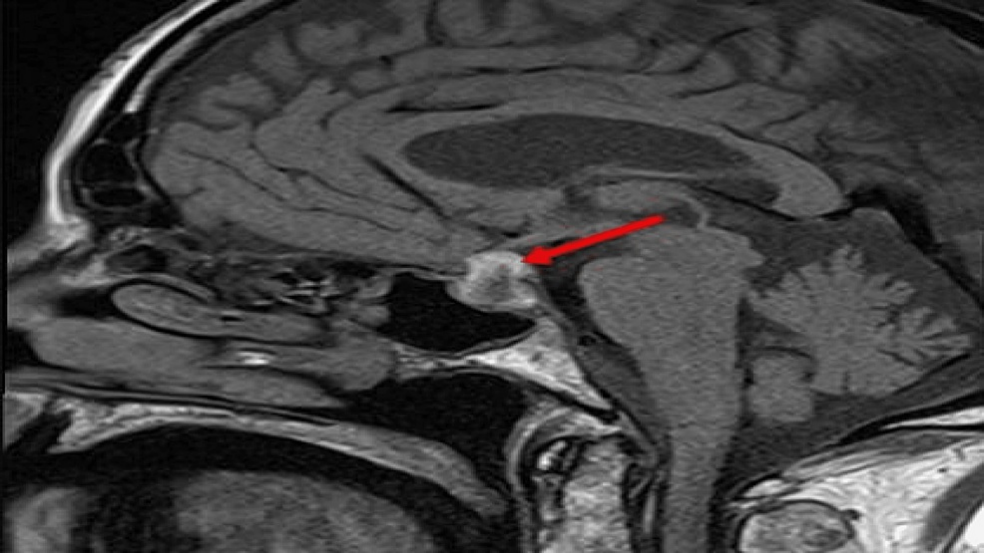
MRI of the brain Sagittal T1 pre-contrast reveals a pituitary region mass with suprasellar extension. It has an intrinsic high T1 signal peripherally, in keeping with blood (red arrow).
